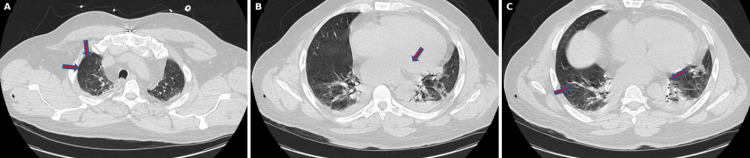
Computed tomography of the chest (lung windows). (A) Faint right-upper-lobe ground-glass and mosaic attenuation. (B) Lingular consolidation silhouetting the left heart border. (C) Dense, dependent bibasilar consolidation. Arrows indicate the findings.
